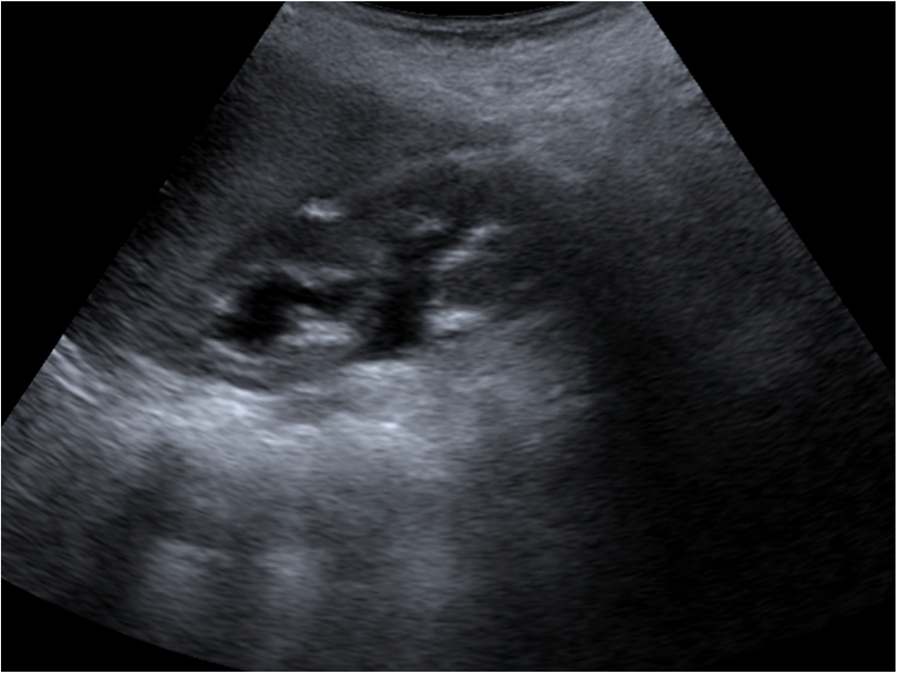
Ultrasound image 30 months after surgery
